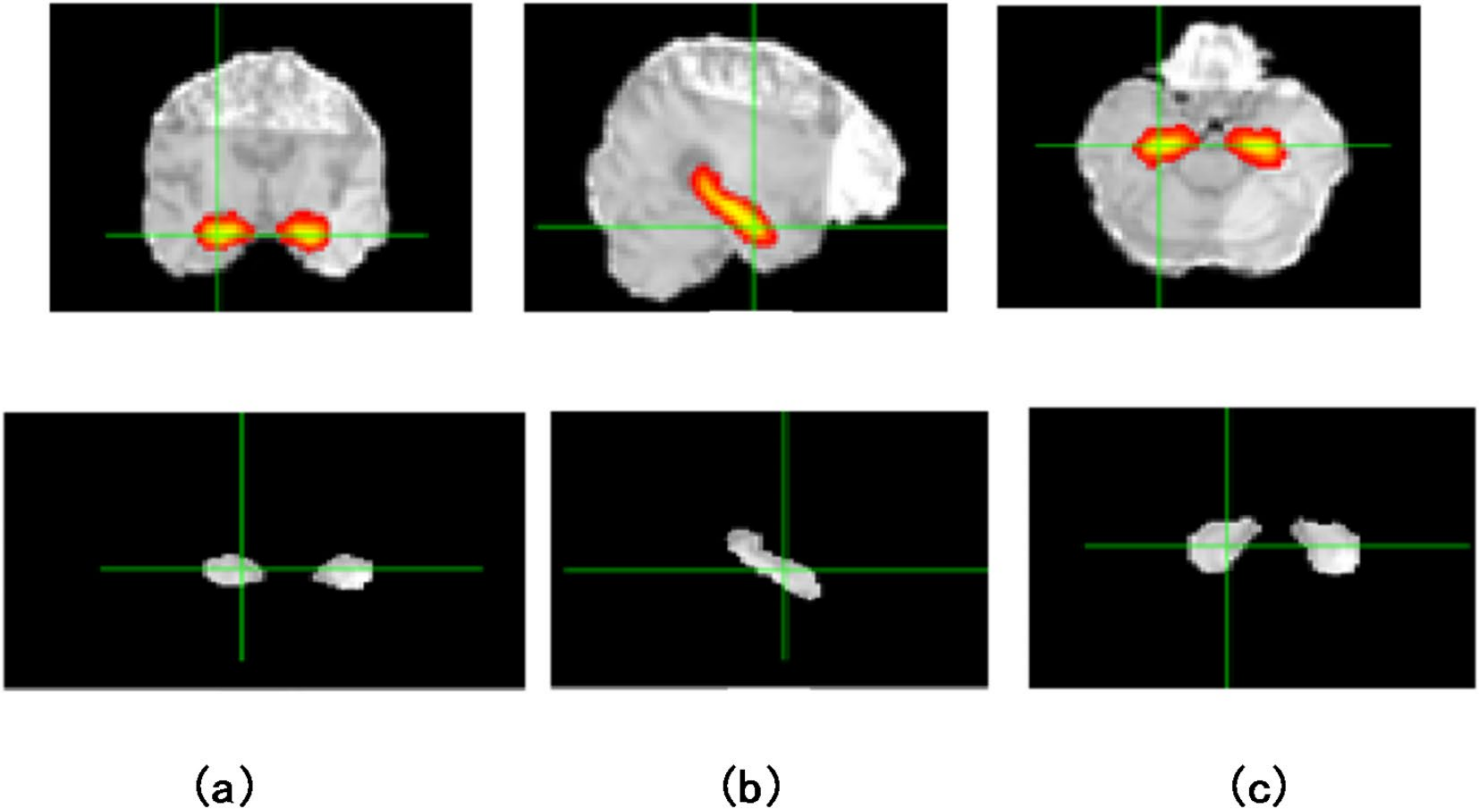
Hippocampus ROI selection in three planes of an MRI slice and Segmented Hippocampus region for (a) Axial, (b) Sagittal and (c) Coronal planes.
